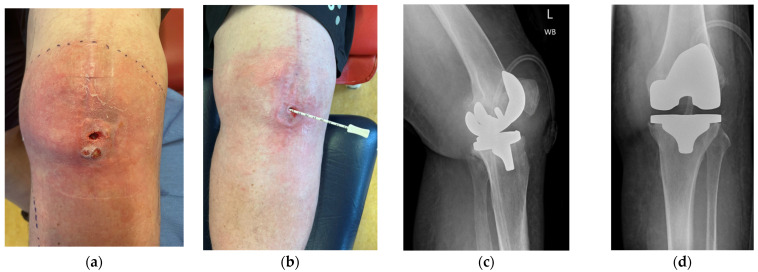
(a,b) Case 2 clinical findings at presentation and (c,d) radiographic findings during treatment.
